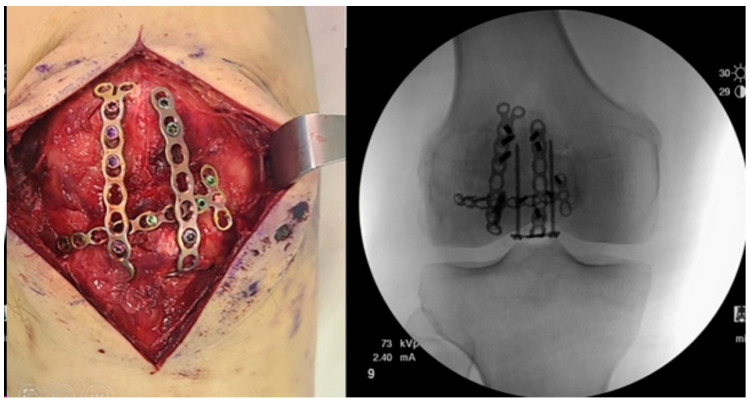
Depicts the fixation of a comminuted patellar fracture using locking compression miniplates and depicts the radiographic image. Copyright BMC Musculoskeletal Disorders [74].
